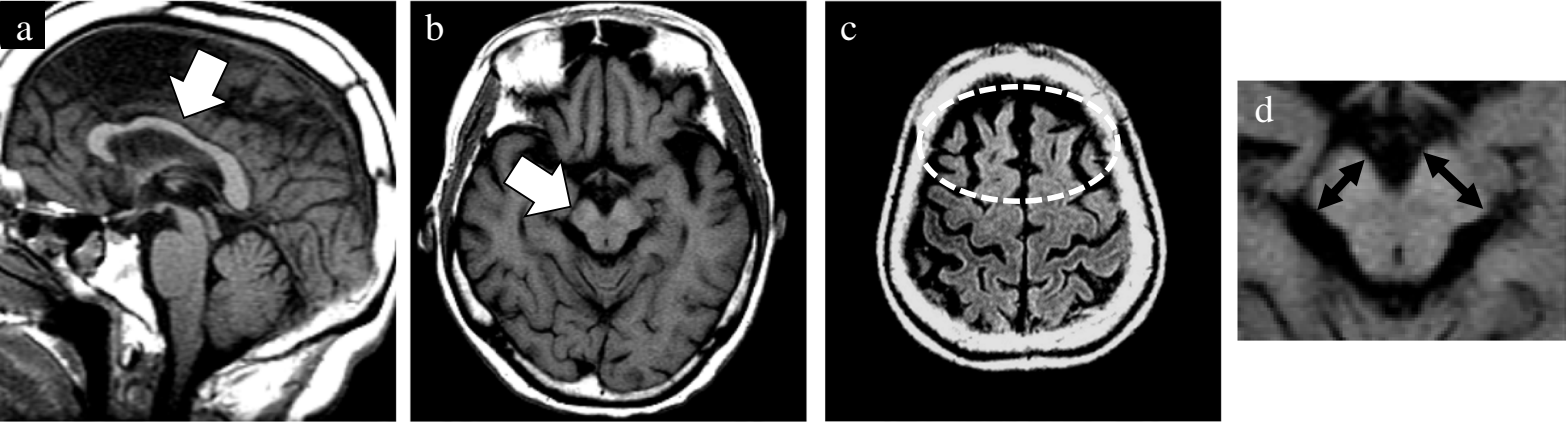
A representative case of radiologically typical CBD. This 69-year-old female patient obtained an antemortem clinical diagnosis of AD. After the postmortem pathological examination, it was proved that main pathology of this patient was CBD. MRI was performed one year after the symptom onset. In contrast to mild atrophy of the callosal body (arrow, a), sagittal T1WI showed relatively preserved midbrain. Axial T1WI and FLAIR image showed right dominant asymmetric atrophy of the cerebral peduncle and frontal lobe (arrow, b). Additionally, mild SWMH was detected in the frontal lobe at high convexity (circle, c). A magnified view was exhibited to emphasize the asymmetry of the short axis in the cerebral peduncles (double sided arrows, d). a, sagittal T1WI; b, axial T1WI; c, axial FLAIR image; AD, Alzheimer’s disease; CBD, corticobasal degeneration; FLAIR, fluid-attenuated inversion recovery; SWMH, subcortical white matter hyperintensity; T1WI, T1-weighted image
